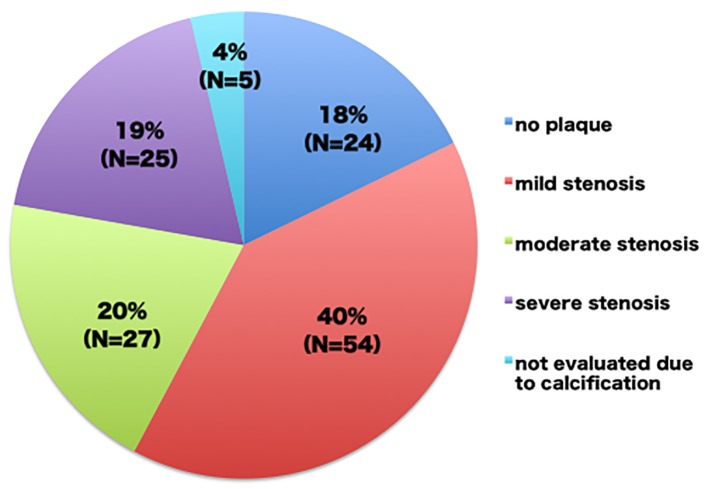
The coronary computed tomography angiography findings in the referred patients with multiple coronary risk factors and carotid atherosclerosis.
